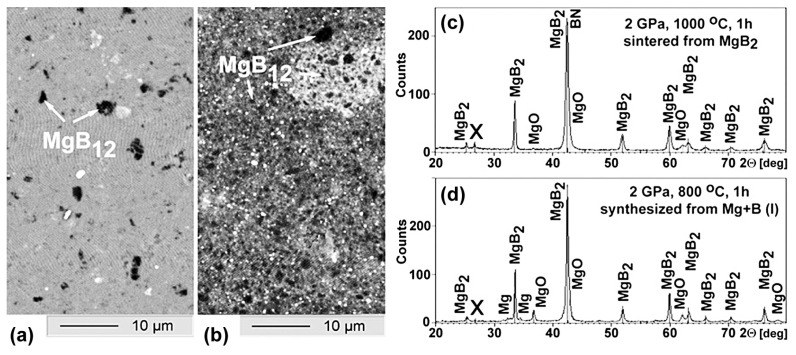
(a,b)—Sample structures obtained by SEM in COMPO (compositional) contrast: (a)—Sample sintered from MgB_2_ (Type VI) under 2 GPa at 1000 °C for 1 h; bright small zones in (a) seem to be inclusions (containing O, Zr, Nb, and possibly ZrO_2_) appearing due to milling of initial MgB_2_. (b)—Structure of sample synthesized from Mg(I):2B(I) under 2 GPa at 800 °C. (c,d)—X-ray patterns of these samples, respectively [109].
